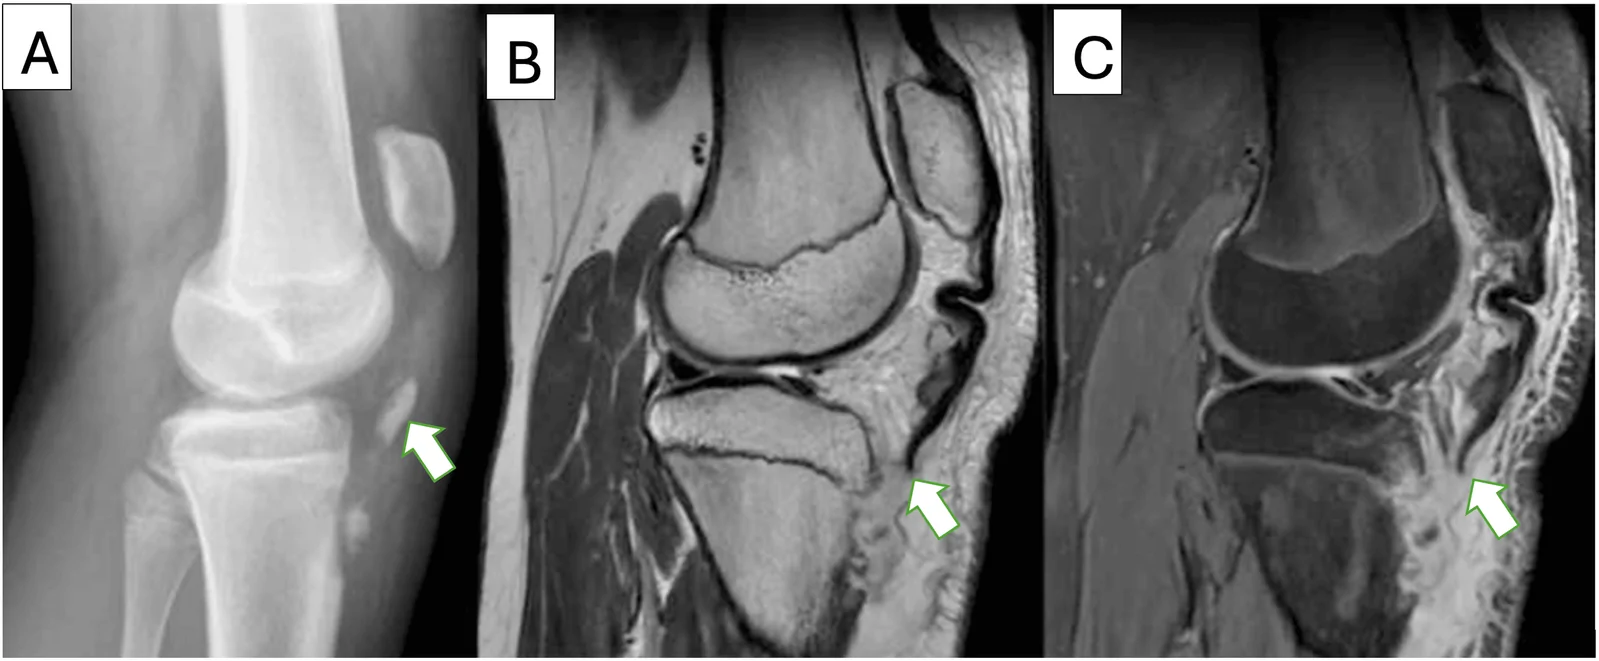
Preoperative lateral radiograph and sagittal magnetic resonance image of case 3. (A) Lateral radiograph, (B) sagittal T1-weighted MRI, and (C) sagittal fat-suppressed T2-weighted MRI demonstrating an Ogden type IIB tibial tubercle fracture without complete rotational displacement of the fragment. The distal patellar tendon avulsion (arrows) is evident on MRI despite the absence of fragment rotation, demonstrating that this radiographic sign is not universally present in bifocal extensor mechanism injuries.
